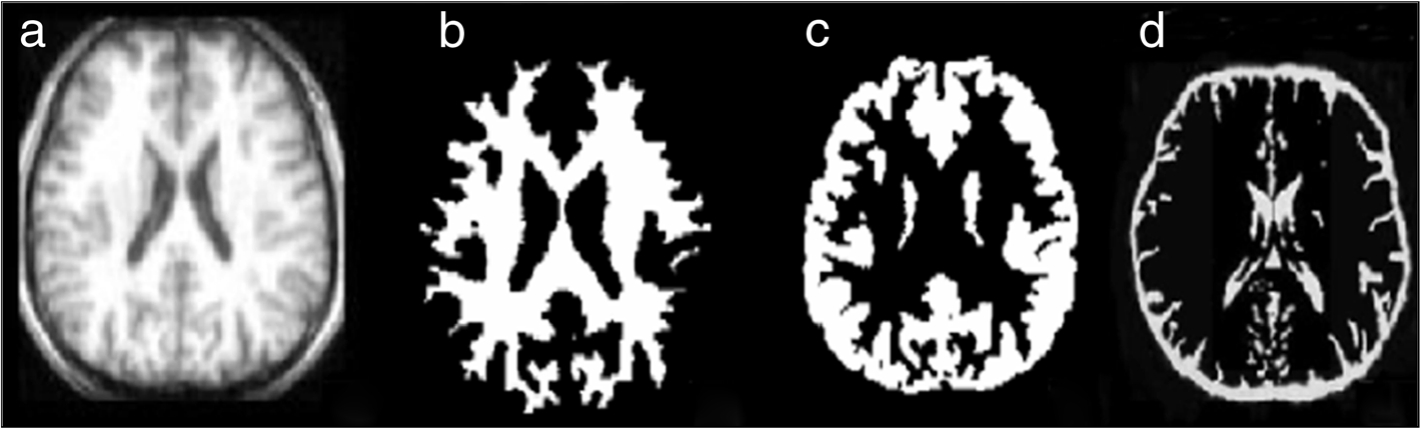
Proposed algorithm applied to real database. From left to right; a brain MRI slice of IBSR Database, Estimated WM, Estimated GM, Estimated CSF.
